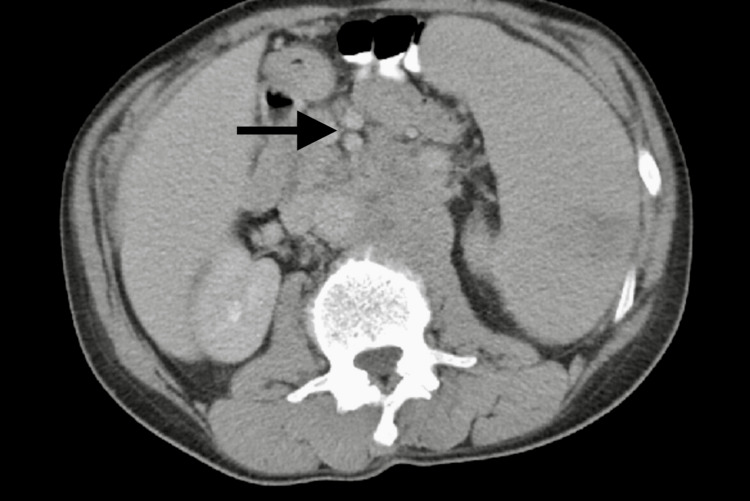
CT abdomen and pelvis showing evidence of abdominal lymphadenopathy
